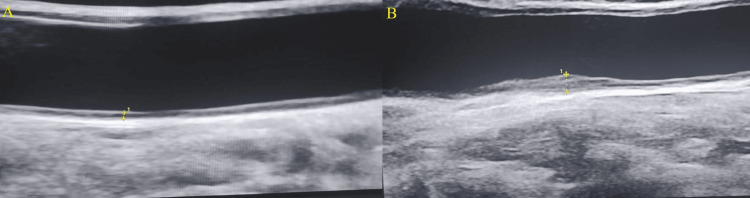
A sample USG image showing CIMT measured in control and case (A) Control: 53-year-old male patient (yellow markings depicting the CIMT measured at 0.30 mm). (B) Case: 43-year-old male patient (yellow markings depicting CIMT measured at 1.80 mm) USG: ultrasonography; CIMT: common carotid artery intima-media thickness
